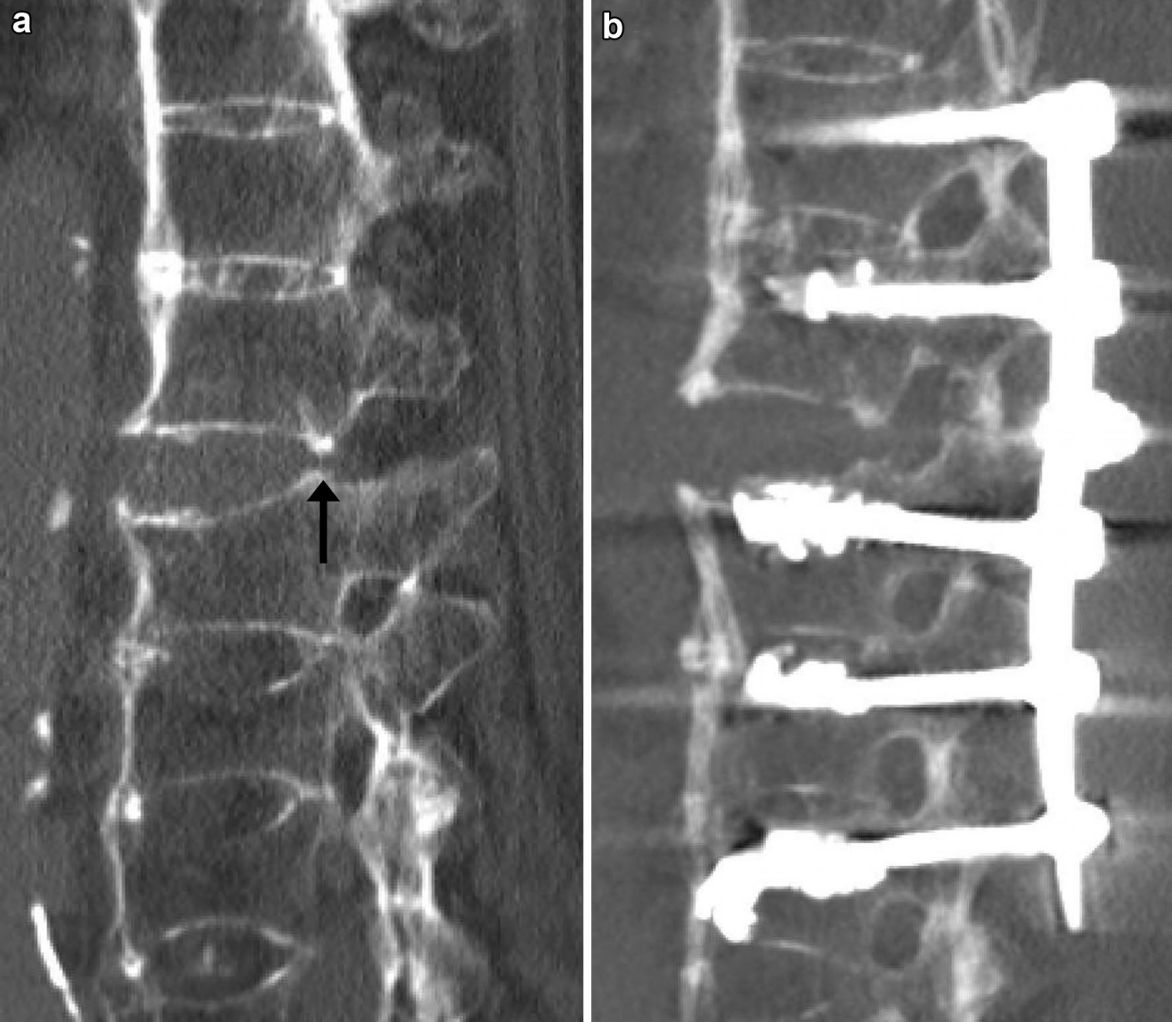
81-year-old male, hyperextension injury of Th12/L1, 13° of lordotic angulation in the pre-operative CT scan (a). Postoperative CT scan prior to mobilisation after cement-augmented open rigid posterior instrumentation. No relevant intraoperative reduction of lordotic angulation (b)
